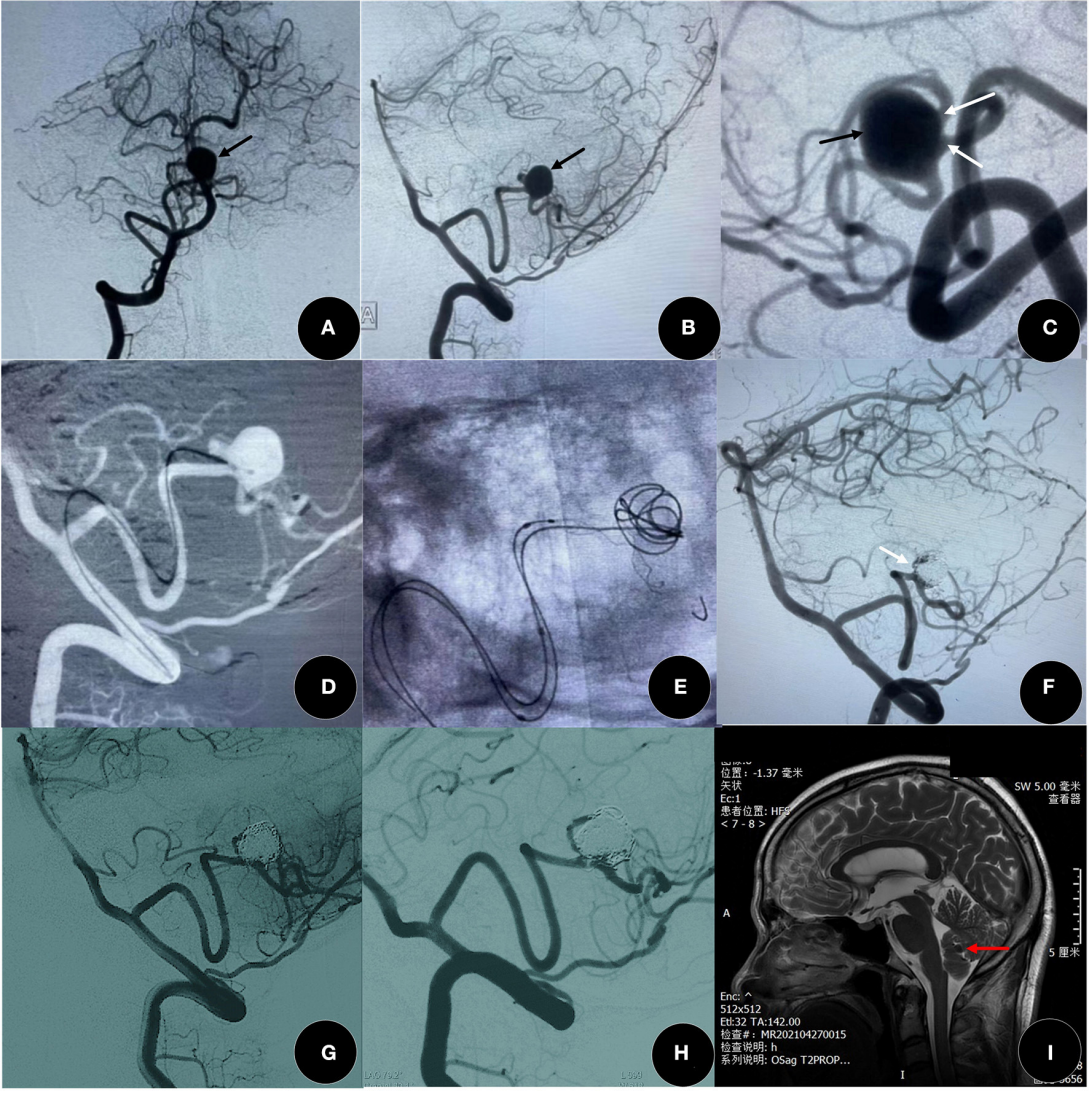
A 51-year-old woman presented with a headache for 1 year. (A–C) The angiographic result showed a distal posterior inferior cerebellar artery (PICA) aneurysm (black arrow) with two side branches (white arrow). (D–G) The aneurysm was in good occlusion (RROC 2) by Leo plus baby (2.5 mm × 18 mm) assisted coiling, and two branches were well-protected (white arrow). (G,H) The degree of occlusion increased to complete occlusion (RROC 2) at 9 months after surgery. (I) There is no new cerebral infarction after the confirmation of SAC by MRI, and red arrow indicates coils. SAC, stent-assisted coiling.
